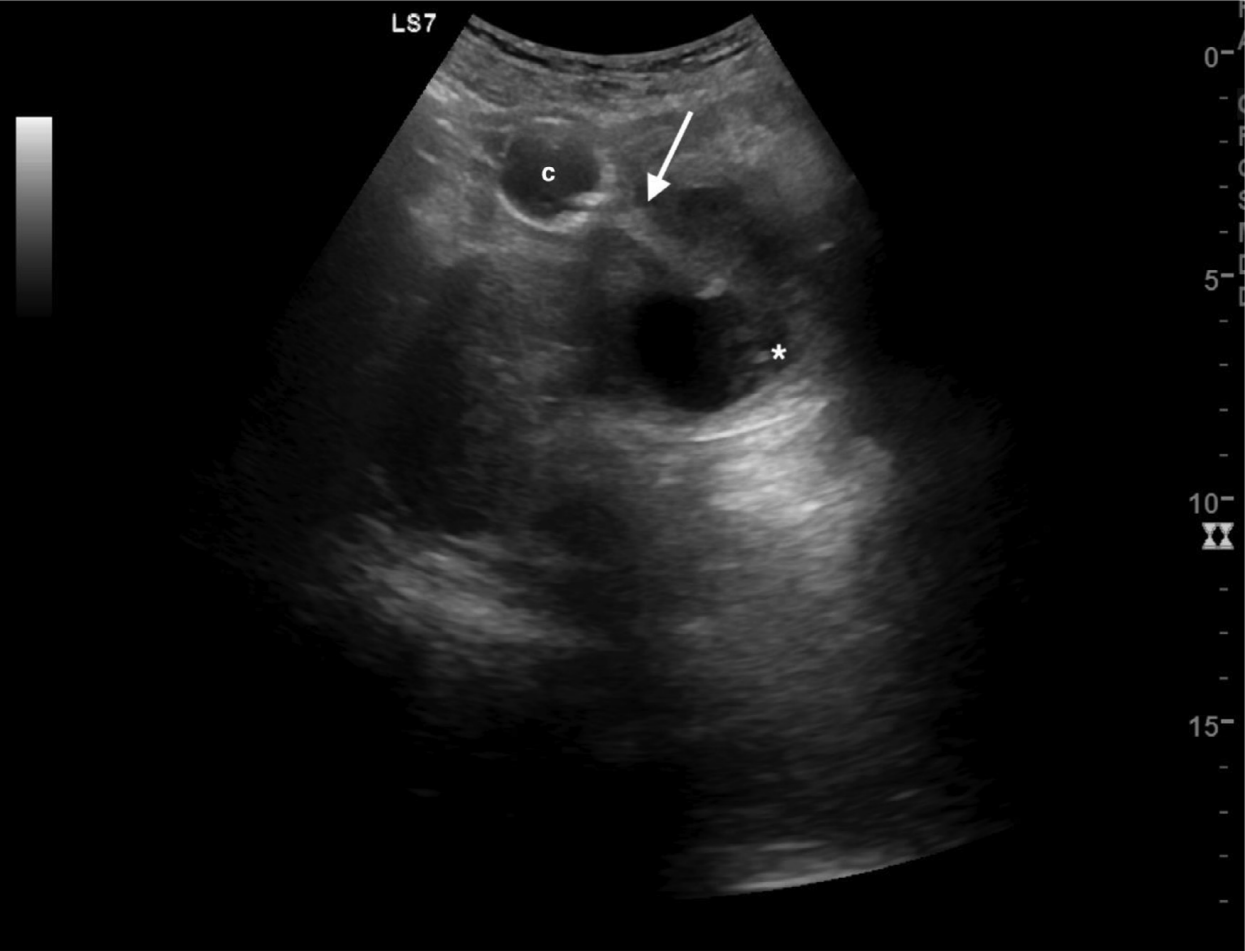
Transabdominal ultrasound after emptying the bladder. The catheter balloon (c) was visible on the upper pole of the lesion, the hyperechoic catheter line run within the midline septum (white arrow). The fluid content is inhomogeneous (white asterix).
